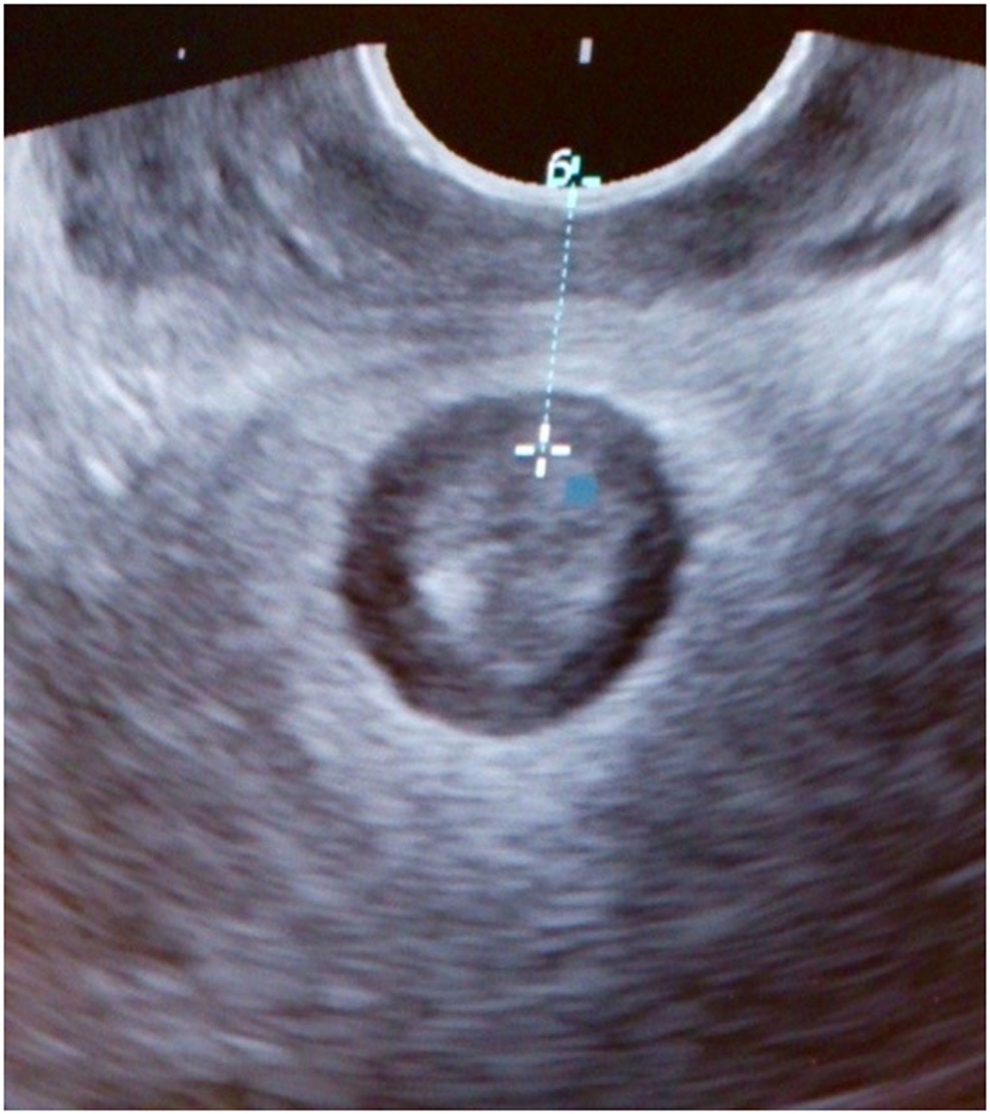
Perineal ultrasound image, obtained with a vaginal probe showing measurement of the anovaginal distance
